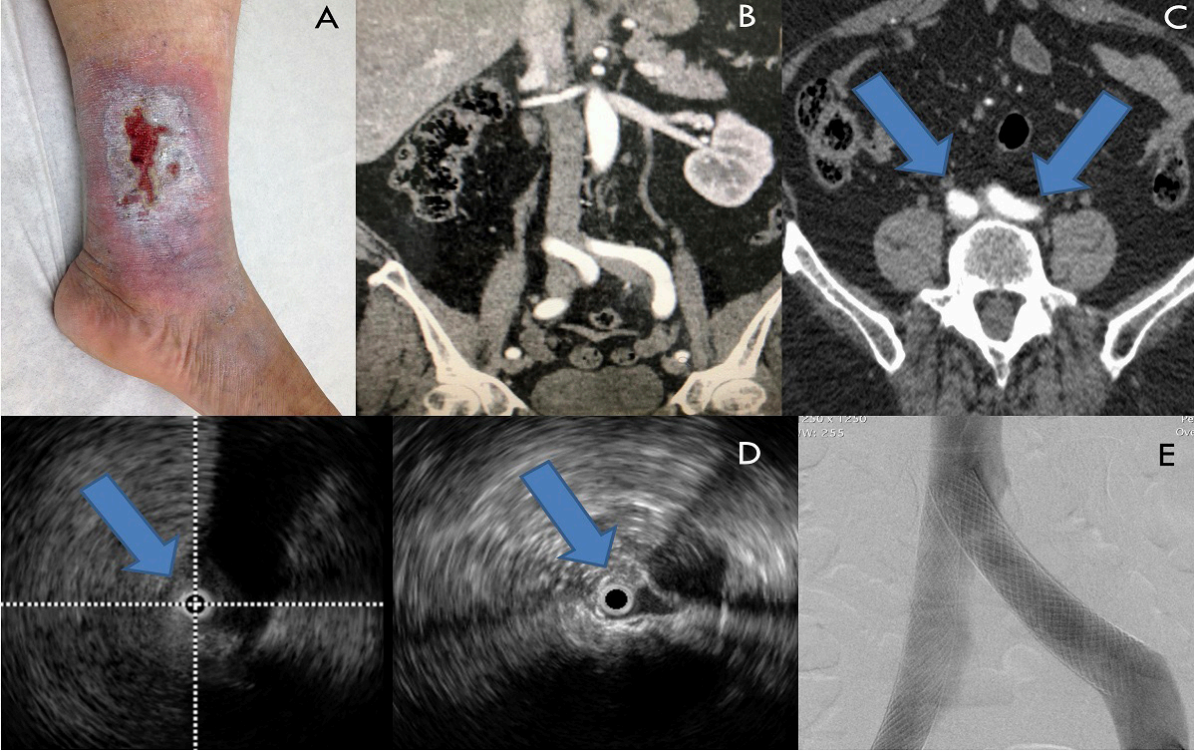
Angioplasty with bilateral stenting in a patient with clinical, etiological, anatomic, and pathophysiological classification (CEAP) classification grade C6, with a severe obstruction compromising the iliac veins bilaterally: (A) Unilateral venous ulcer (chronic venous insufficiency, CEAP C6S); Presence of severe bilateral iliac compression: (B, C) Angiotomography; (D) intravascular ultrasound, confirming severe bilateral obstruction (>50%); (E) Satisfactory result with stent deployment using the double-barrel technique.
